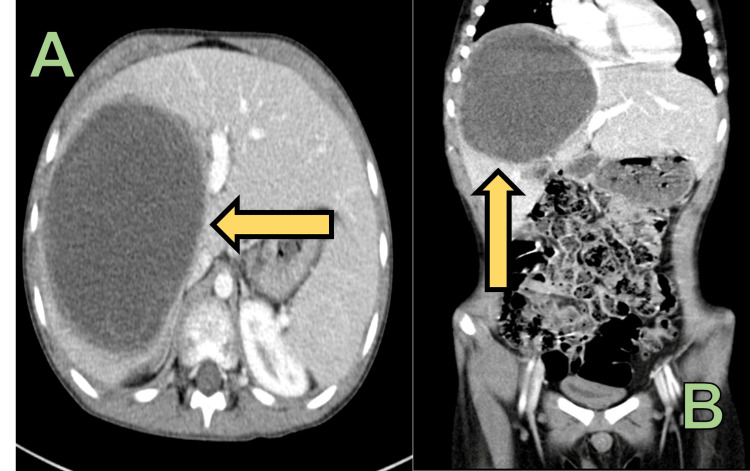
CT abdomen with IV contrast showing significant interval decrease in the size of the right hepatic lobe mass to 11.4 × 10.8 × 12.6 cm A: Axial view; B: Coronal view
